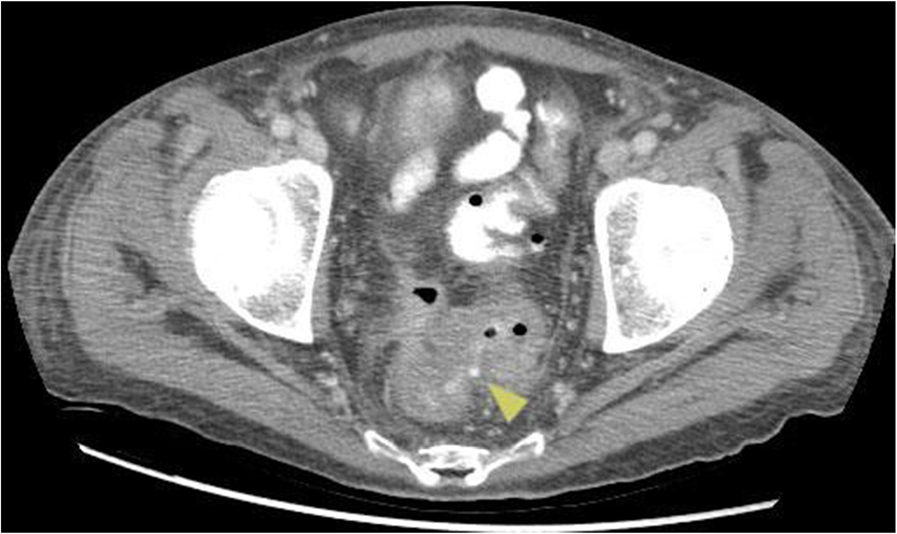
Computed tomography pelvis scan shows focal rectosigmoid thickening with small fistula from sigmoid to rectum (*yellow arrow*) in the region of inflamed diverticula suggesting complicated diverticulitis. The fistula formation suggests an element of chronicity
